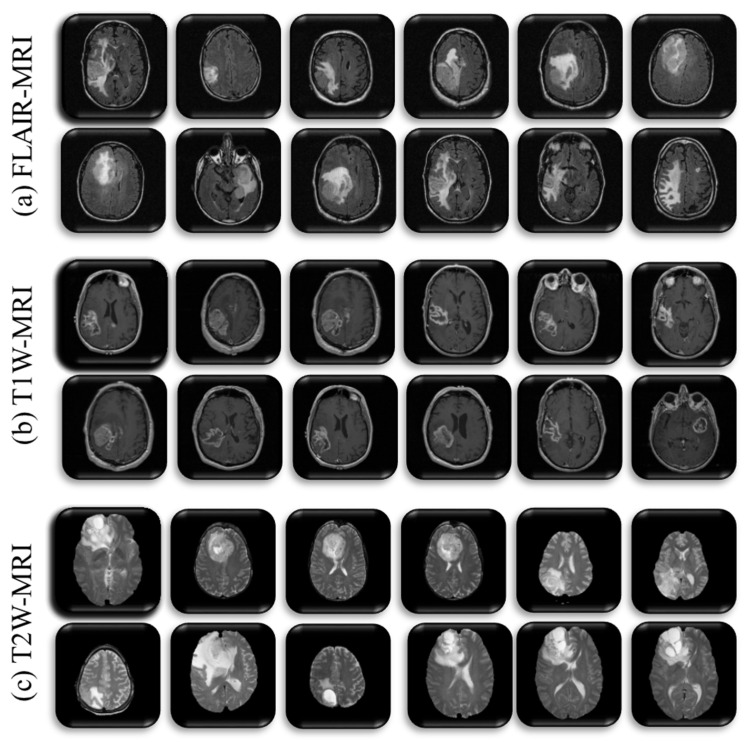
Sample images of three MRI sequences dataset, (a) T1W, (b) T2W, (c) FLAIR.
